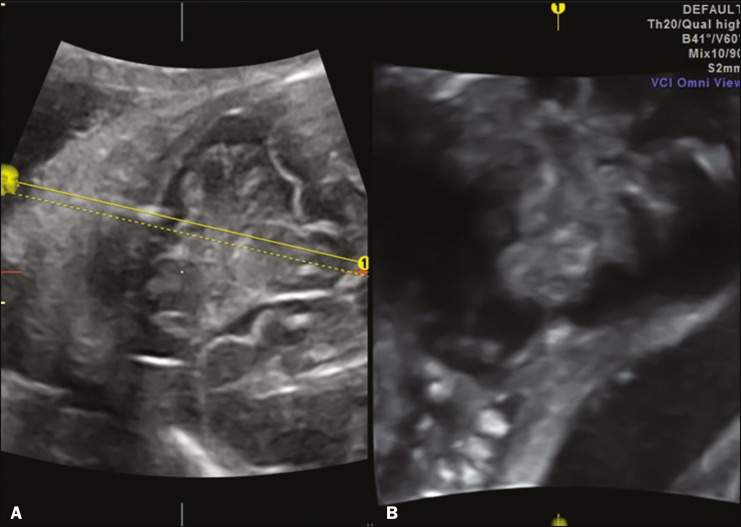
3D ultrasonography of the posterior fossa at 28 weeks of gestation, which allows multiplanar evaluation (OmniView). A: Axial acquisition in the transcerebellar plane. B: Reconstruction in the sagittal plane (OmniView) of the posterior fossa.
